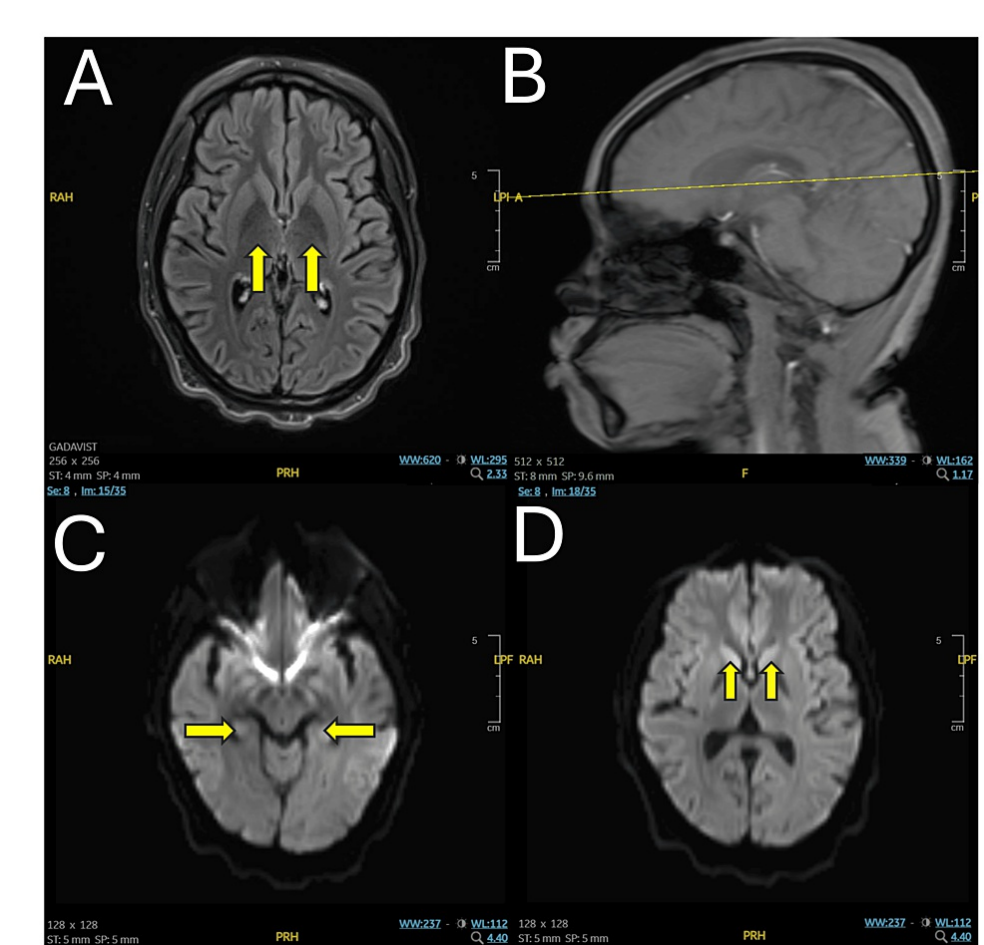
MRI brain (A) Axial view T2 flair, yellow arrows indicating posterior limb of the internal capsule, (B) sagittal view T2 flair, yellow cross-section of view from panel A, (C) axial view diffusion tensor imaging, yellow arrows indicating parahippocampal gyri, (D) axial view diffusion tensor imaging, yellow arrows indicating caudate nuclei head.
